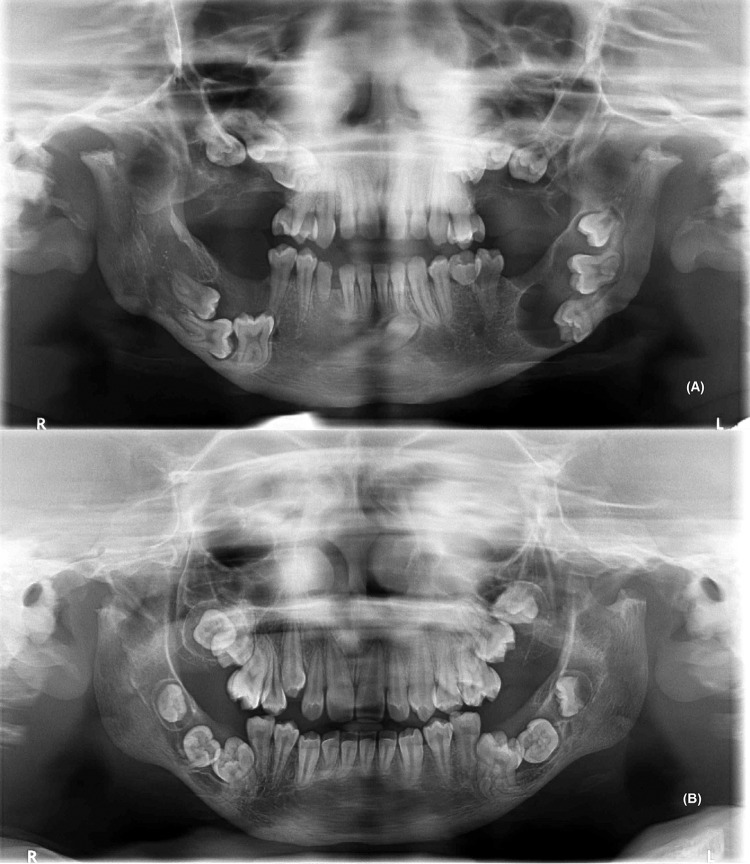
Panoramic radiographs of patients with MPS type VI, aged 19 (A) and 18 (B) years old, showing the presence of extensive maxillomandibular alterations
